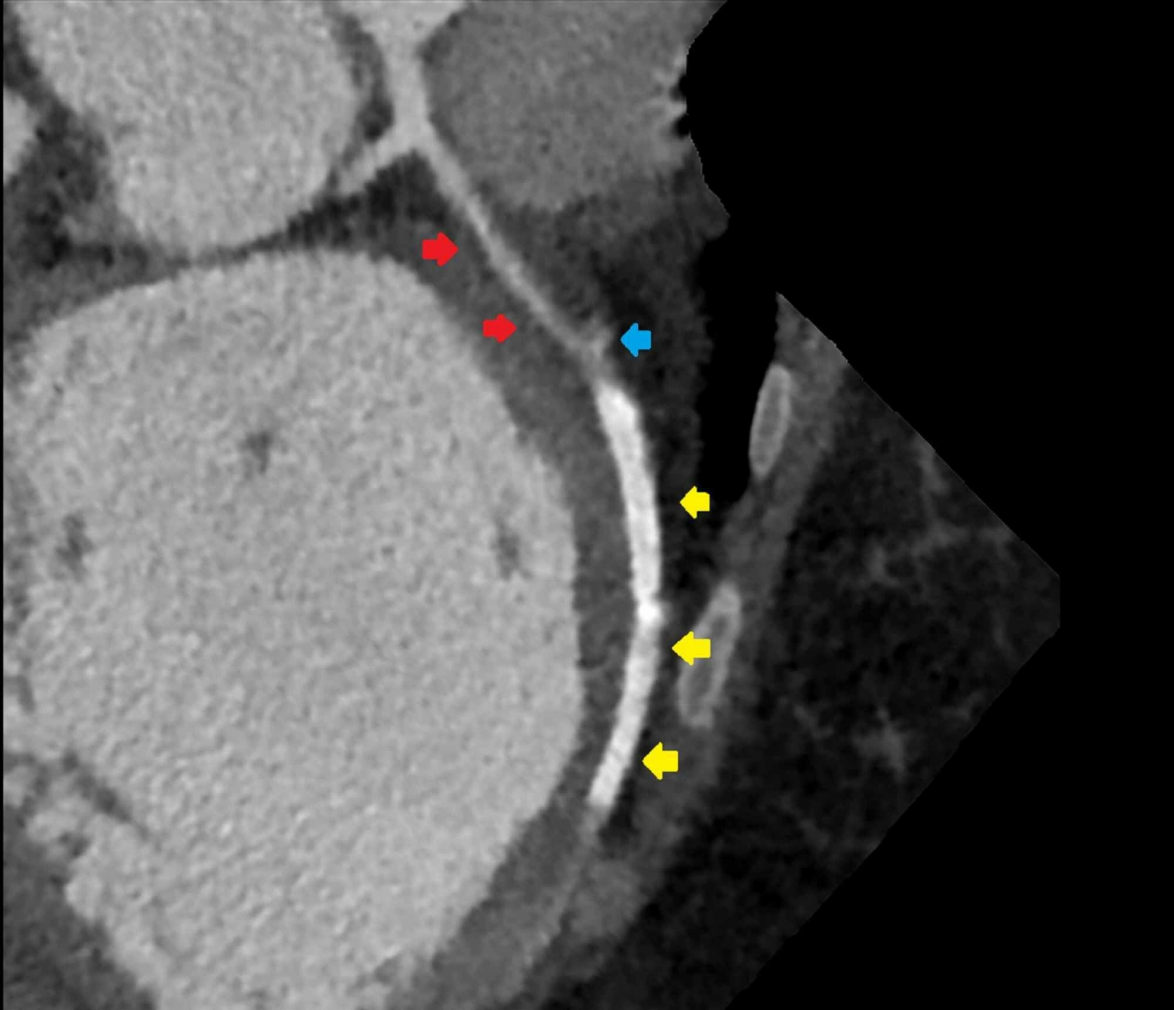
Coronary computed tomography angiography showing proximity of the thrombosed false lumen of dissection to origin of the left main artery (red arrows), proximal left anterior descending artery area of true and false lumen communication (blue arrow), and mid-left anterior descending artery stent (yellow arrows)
